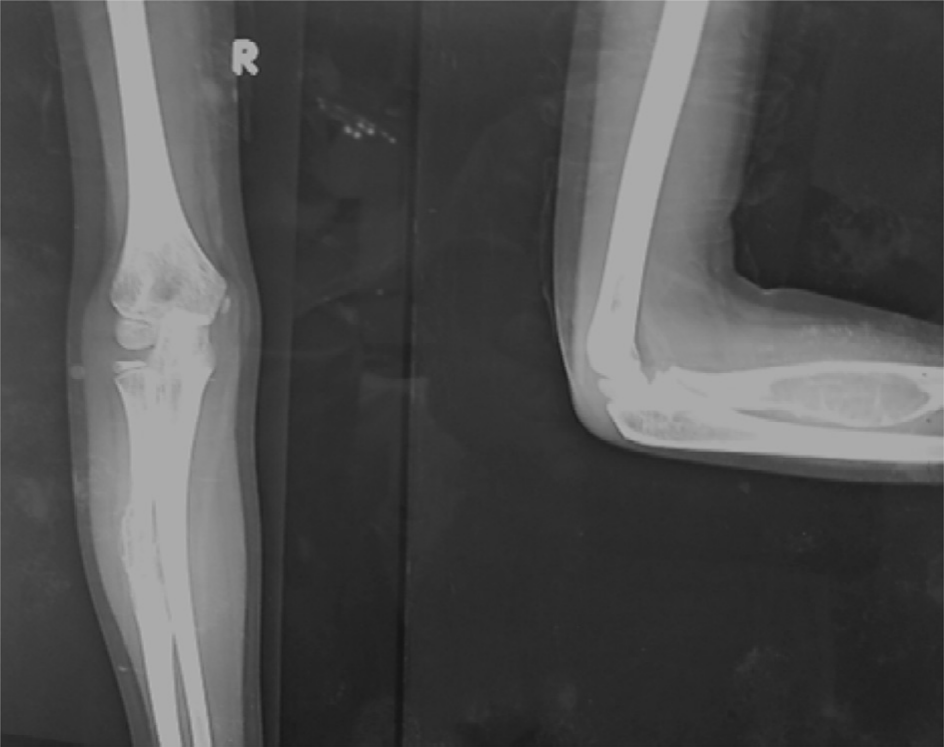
Initial anteroposterior and lateral views of roentgenograph showing an expansile lytic lesion in proximal half of the radius.
